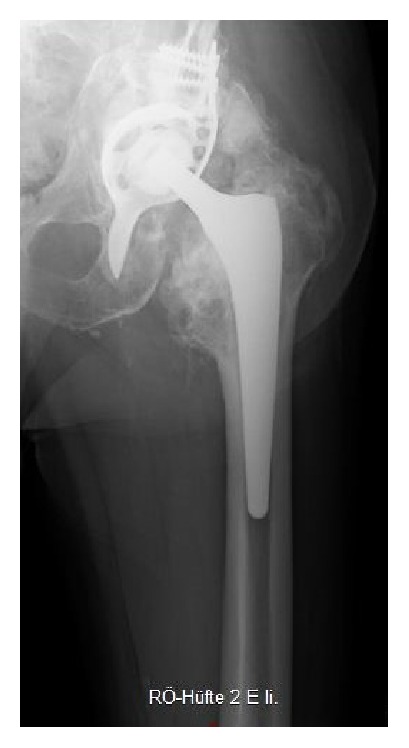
Follow-up X-ray of left hip a.p. two years and eight months postoperatively.
